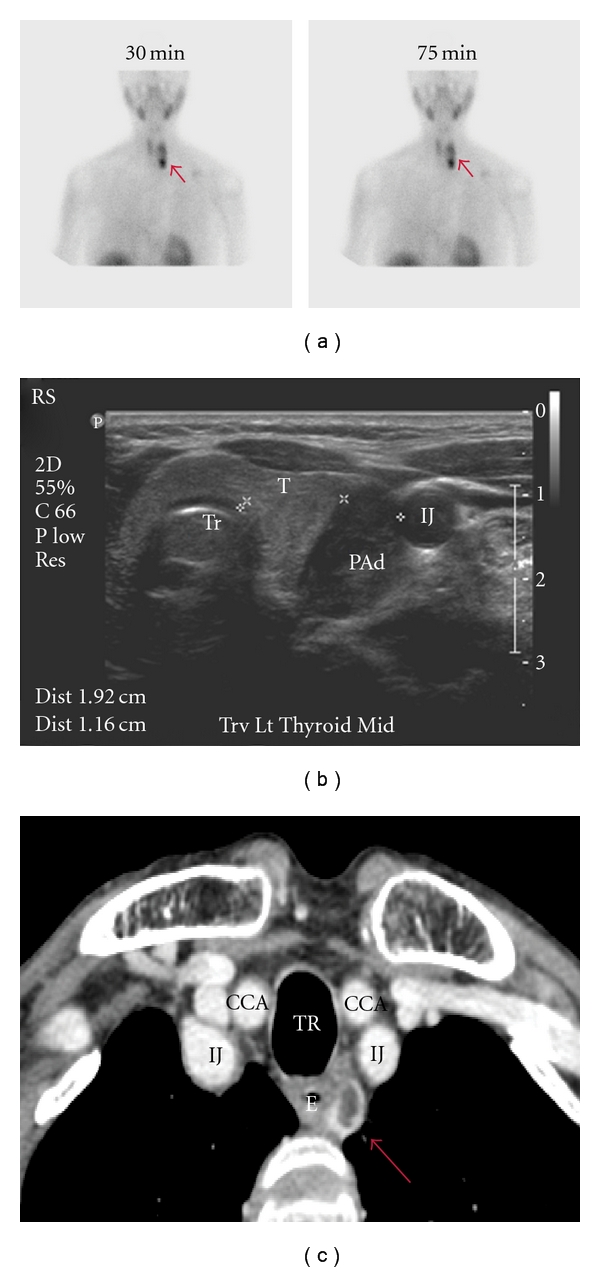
Preoperative imaging in primary hyperparathyroidism. (a) Sestamibi with SPECT displaying focal retention in the left lower position (arrow) after 30 and 75 minutes washout, respectively. (b) Corresponding cervical ultrasound from the same patient showing a large hypoechoic cystic parathyroid adenoma (arrow). The left thyroid lobe (T), trachea (Tr), and left internal jugular vein (IJ) are indicated. (c) Four-dimensional parathyroid CT scan depicting an ectopically positioned left superior paraesophageal parathyroid adenoma (arrow). IJ: internal jugular vein; T: trachea; E: esophagus; CCA: common carotid artery.
